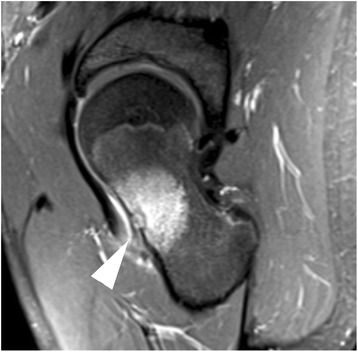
MR of osteoid osteoma. Sagittal T2-w fat saturated MRI image of a 1-cm cortical osteoid osteoma (*white arrowhead*) with prominent bone marrow edema surrounding the left femoral neck lesion. The location of the intraarticular lesion allows for an unobstructed treatment window (i.e., no nerves or other vital structures are present in the ultrasound path)
